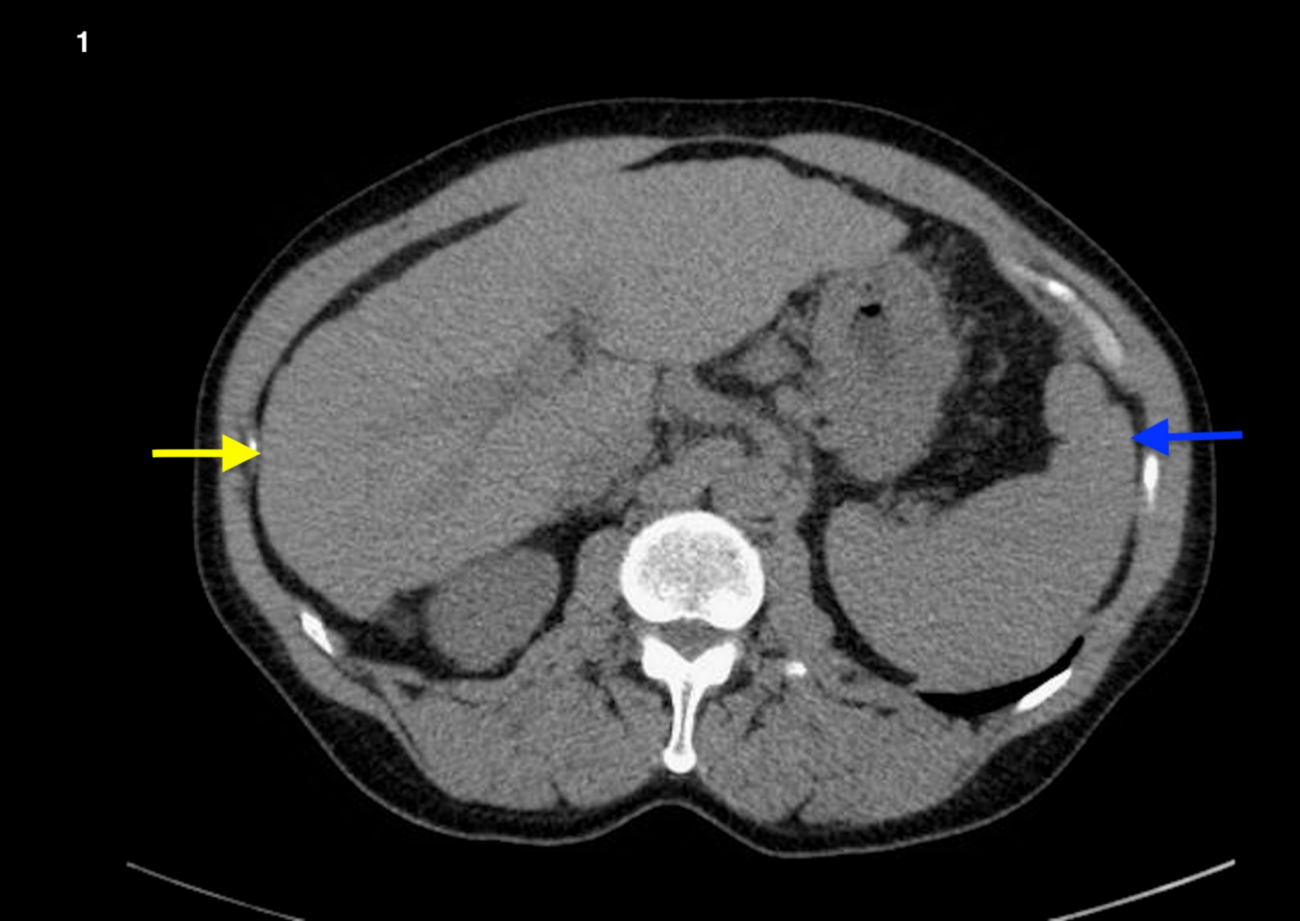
CT of the abdomen of a patient who initially presented with hepatic sarcoidosis and eventually progressed to cirrhosis A shrunken liver with irregular contours (yellow arrow) and an enlarged spleen (blue arrow)
